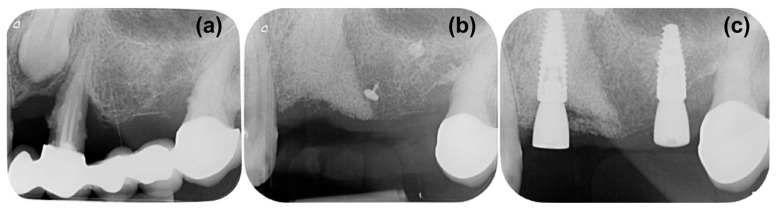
Comparison of periapical X-rays during different phases of the treatment, (a) the initial situation, (b) control X-ray at 8 months, prior to reentry, (c) post operative X-ray after implant placement.
